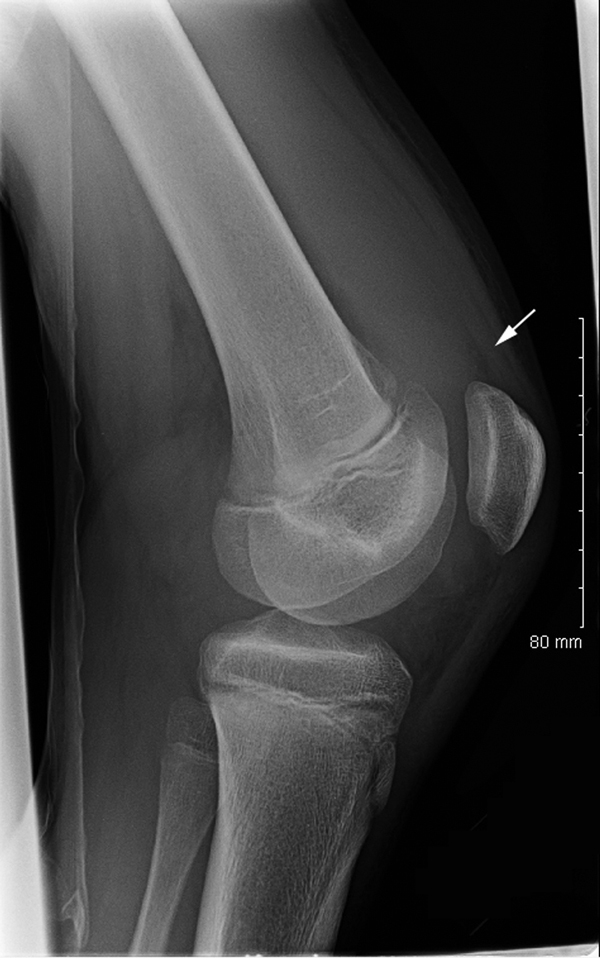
Lateral radiograph of right knee demonstrating suprapatellar effusion without acute osseous injury (arrow).
